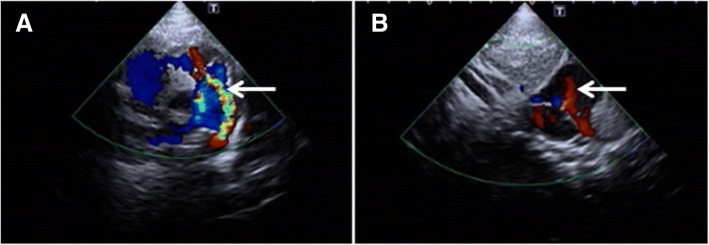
Echocardiography showed (a) a patent ductus arteriosus and (b) patent foramen ovale
